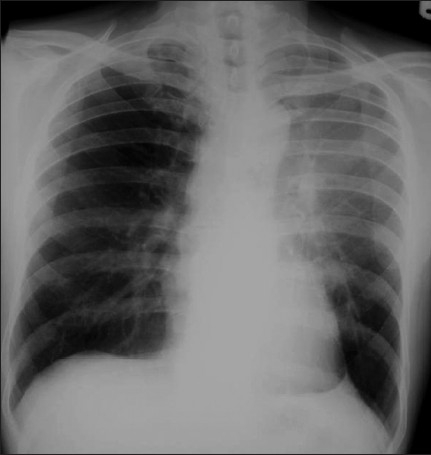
A frontal chest radiograph showing a left–upper-lobe atelectasis. The radiograph reveals hazy opacification of the left hilum, elevation of the left hilum, near-horizontal course of the left main bronchus, posterior leftward rotation of the heart and the Luftsichel or air crescent sign, the name given to the appearance of aerated lung abutting the arch of the aorta, between the mediastinum and the collapsed left upper lobe. An appearance on a lateral radiograph, if available, of the ICU patient may show retrosternal opacity and displacement of the greater fissure anteriorly
